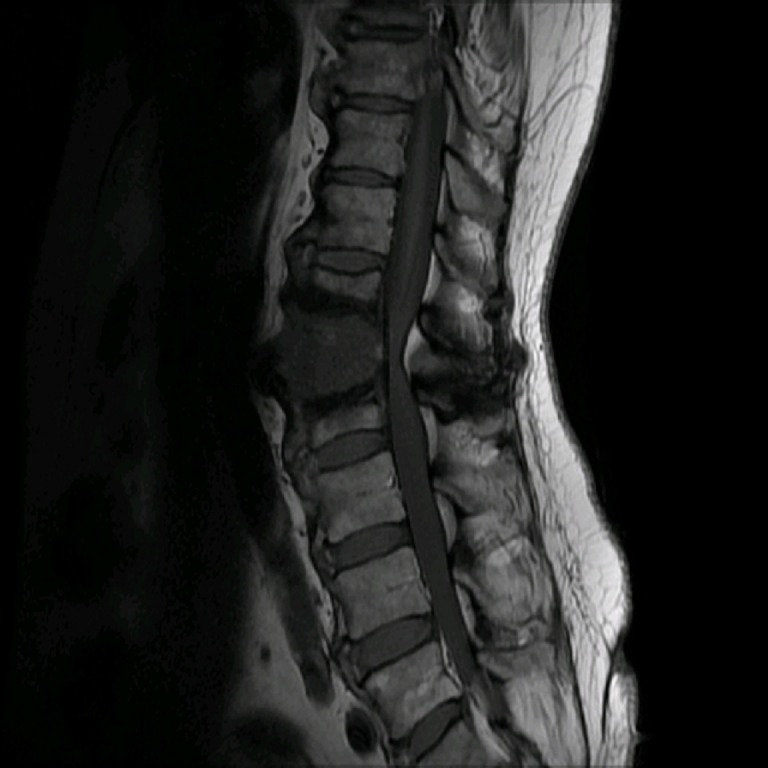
Sagittal T1 MRI This preoperative sagittal T1 weighted MRI without contrast reveals expansion of L1-2 disc space with edema and erosion of adjacent endplates.
